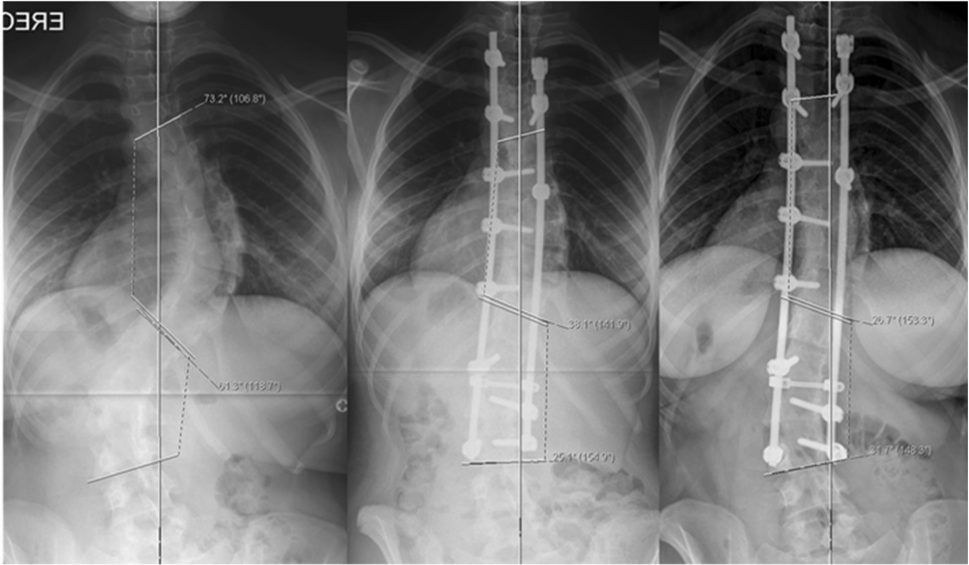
Double structural AIS (*MHHN curve type II*) in 15y/♀ treated by PSF of both curves. 66 % and 50 % correction of main thoracic and compensatory lumbar curves was achieved. The pre-op, immediate post-op and 2.5 years post-op radiographs are shown
